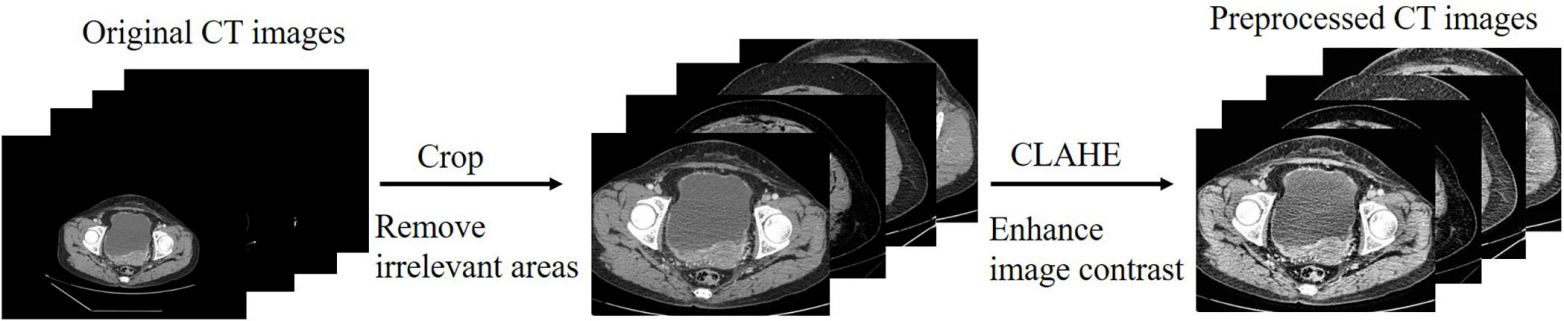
The process of CT image preprocessing.
